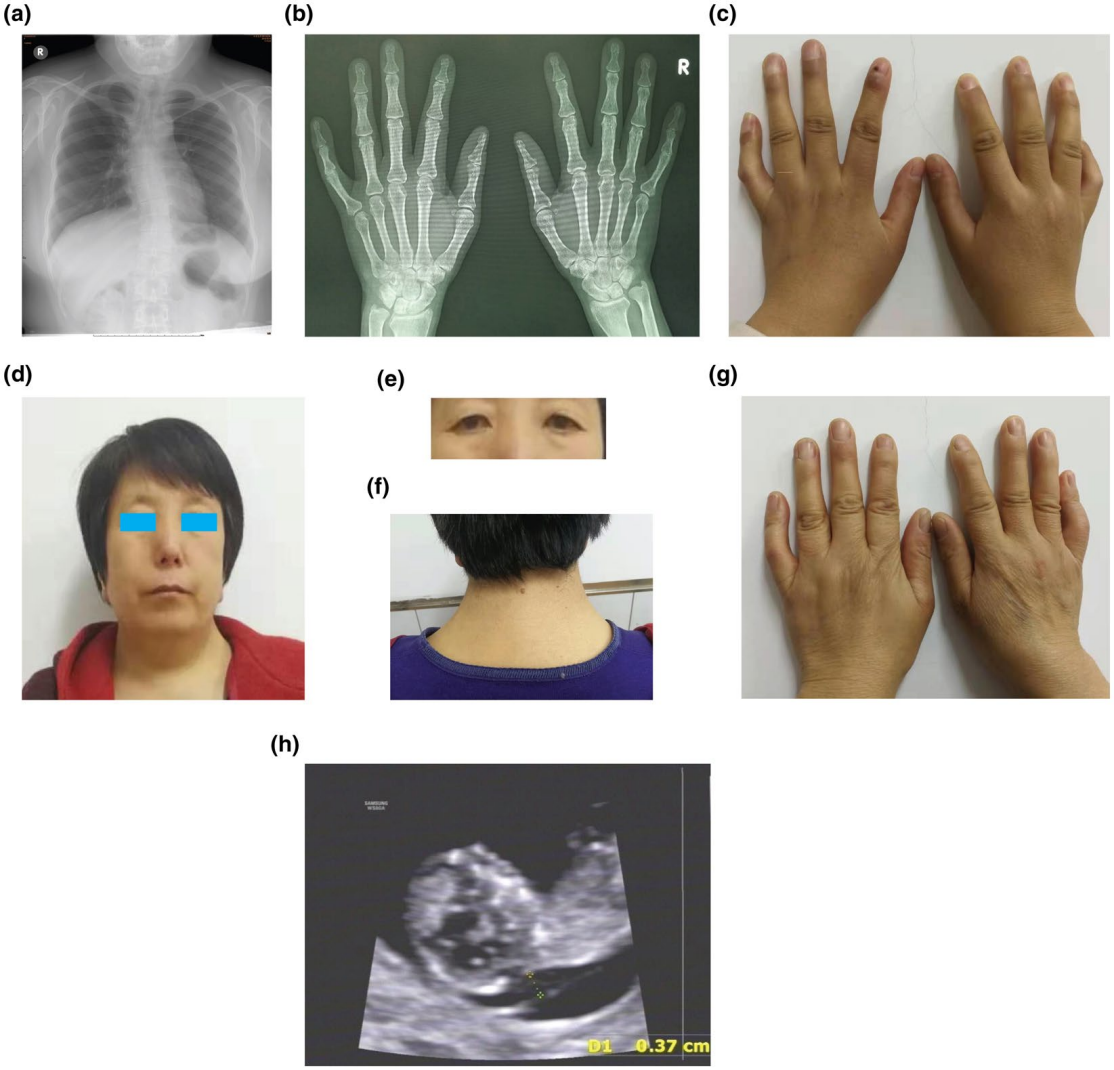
Clinical features and images of the subjects: (a–c) Images of x‐ray chest/hands and hand exterior of the proband. (d–g) Images of the facial features, eyes, neck pterygium, and hand exterior of Ⅲ:2. (h) Ultrasonoscopy of the fetus of the proband at 11^+4d^ gestational weeks
